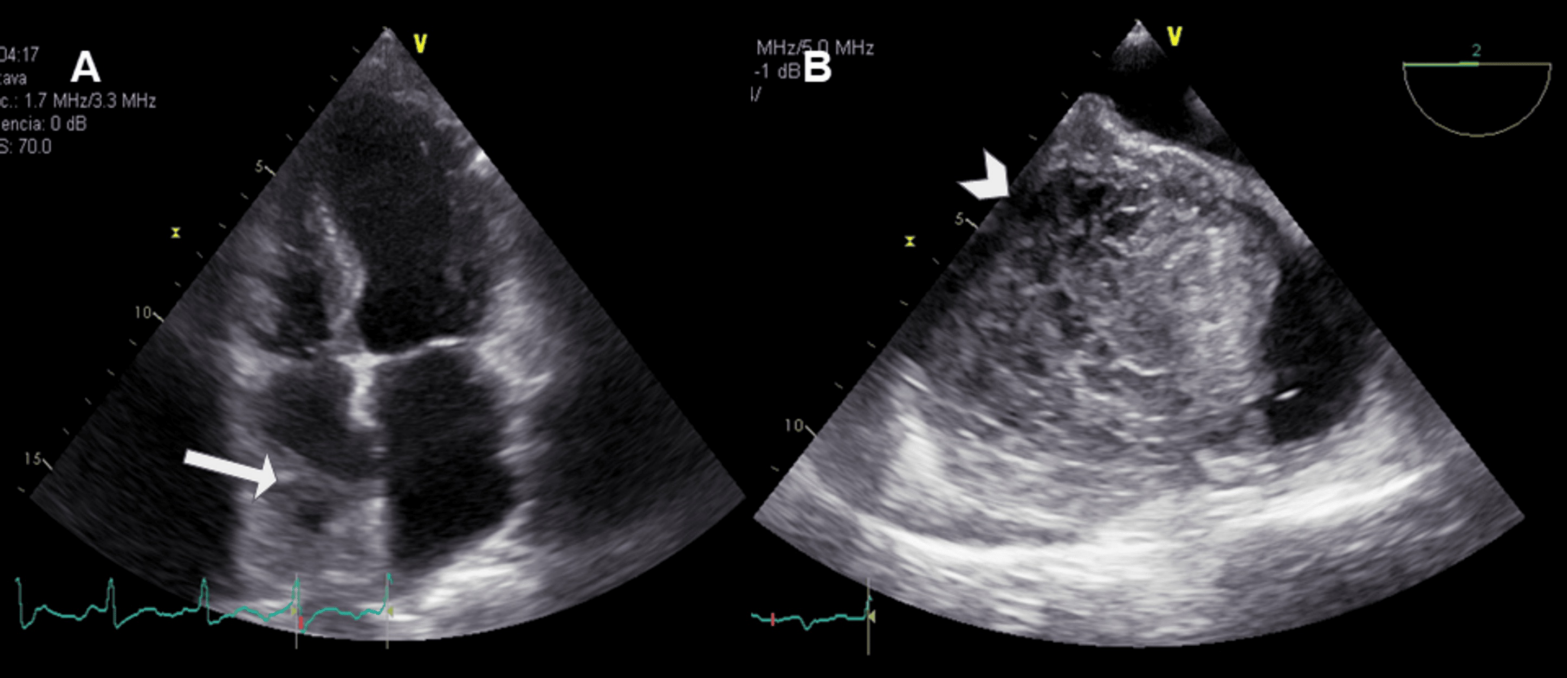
Echocardiographic studies. (A) TTE from an apical four-chamber view showed a mass of intermediate echogenicity occupying the right atrium. There is a hypoechoic center in the mass, suggesting an area of ​​necrosis (arrow). (B) TEE from a mid-esophageal view at the level of the left atrium, confirmed these findings, additionally showing that the mass originated from the lateral and posterior auricular walls with a broad base of implantation and heterogeneous echogenicity (arrow head). TTE: transthoracic echocardiography; TEE: transesophageal echocardiography.
